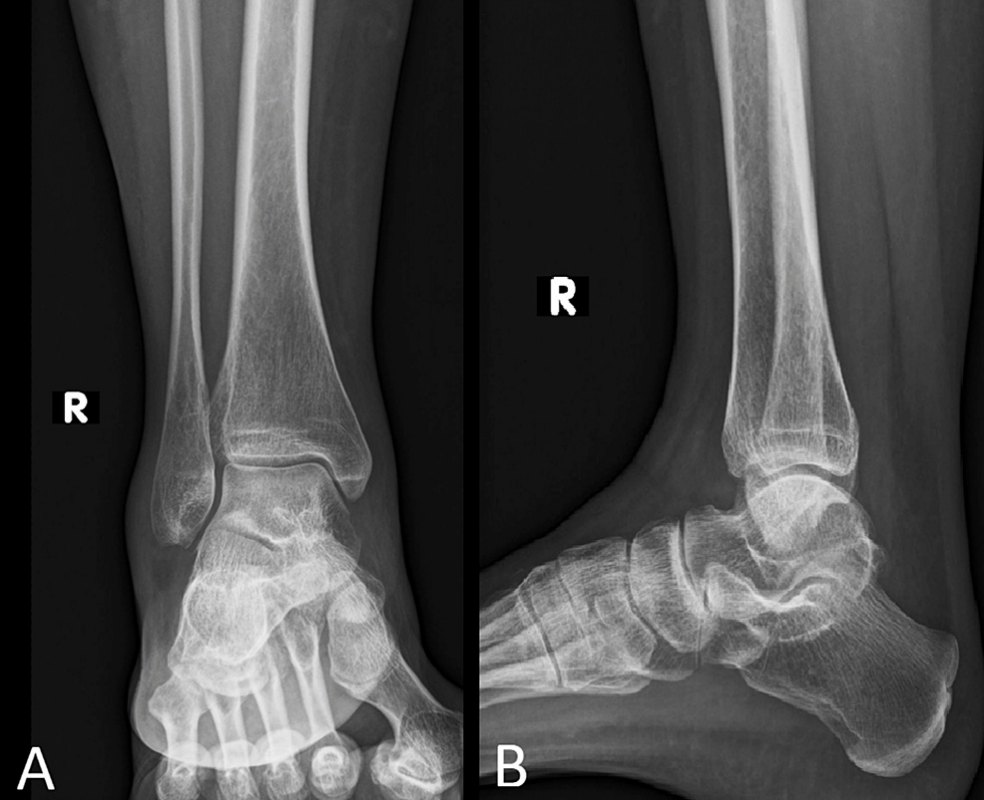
Plain radiographs of the right ankle (A) anteroposterior and (B) lateral views showing no bony abnormalities.
